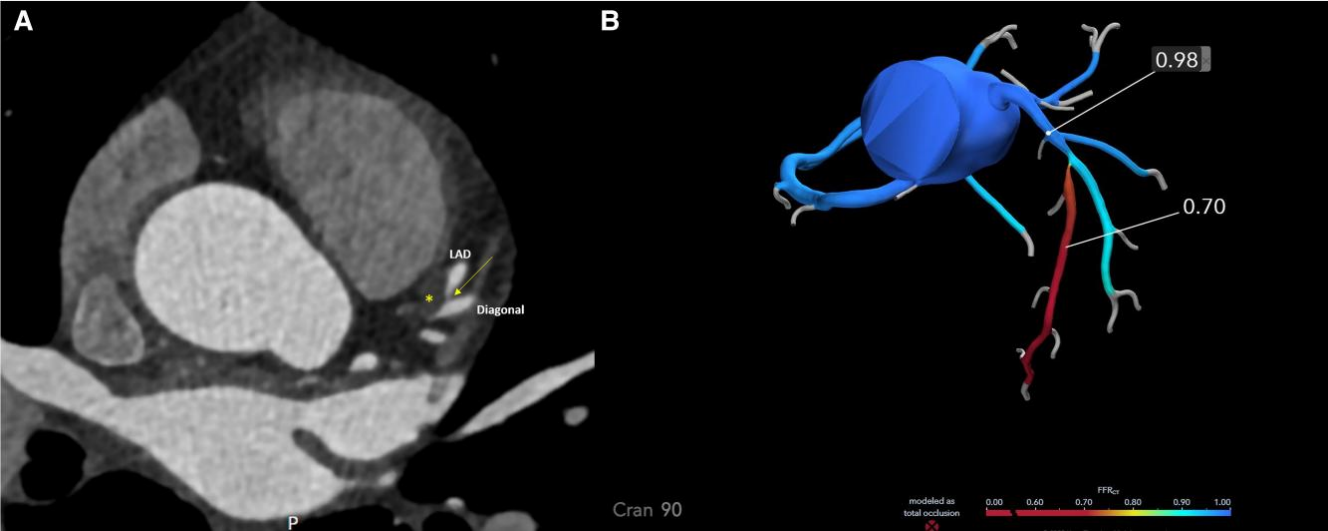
(*A*) Axial CTCA at the level of the bifurcation of the left anterior descending with the large first diagonal branch. The yellow asterisk (*) shows the low-density lipid rich plaque within the proximal and mid-left anterior descending with medina classification of 1,1,0. The yellow arrow shows the very narrow ostium of the mid-left anterior descending. (*B*) CT-derived fractional flow reserve demonstrates a proximal value of 0.98 falling to 0.70 distally across the mid-left anterior descending lesion, confirming haemodynamic significance.
